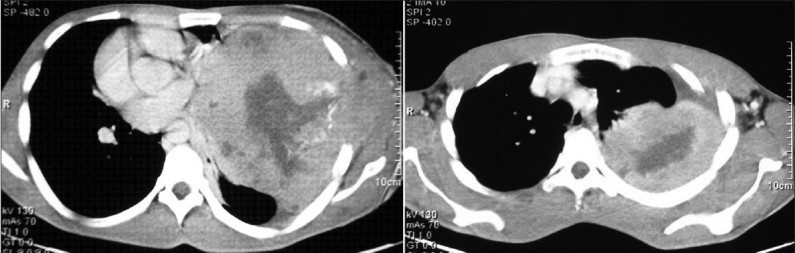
CT scan of the thorax showing large well defined heterogenous mass in the left hemi-thorax. The mass extends peripherally up to the pleura and chest wall, and shows infiltrations into the subcutaneous tissue. The mass also contains areas of low attenuation, corresponding to hemorrhage and necrosis, and extends up to the pleura and the chest wall. There is mediastinal shift to right side
